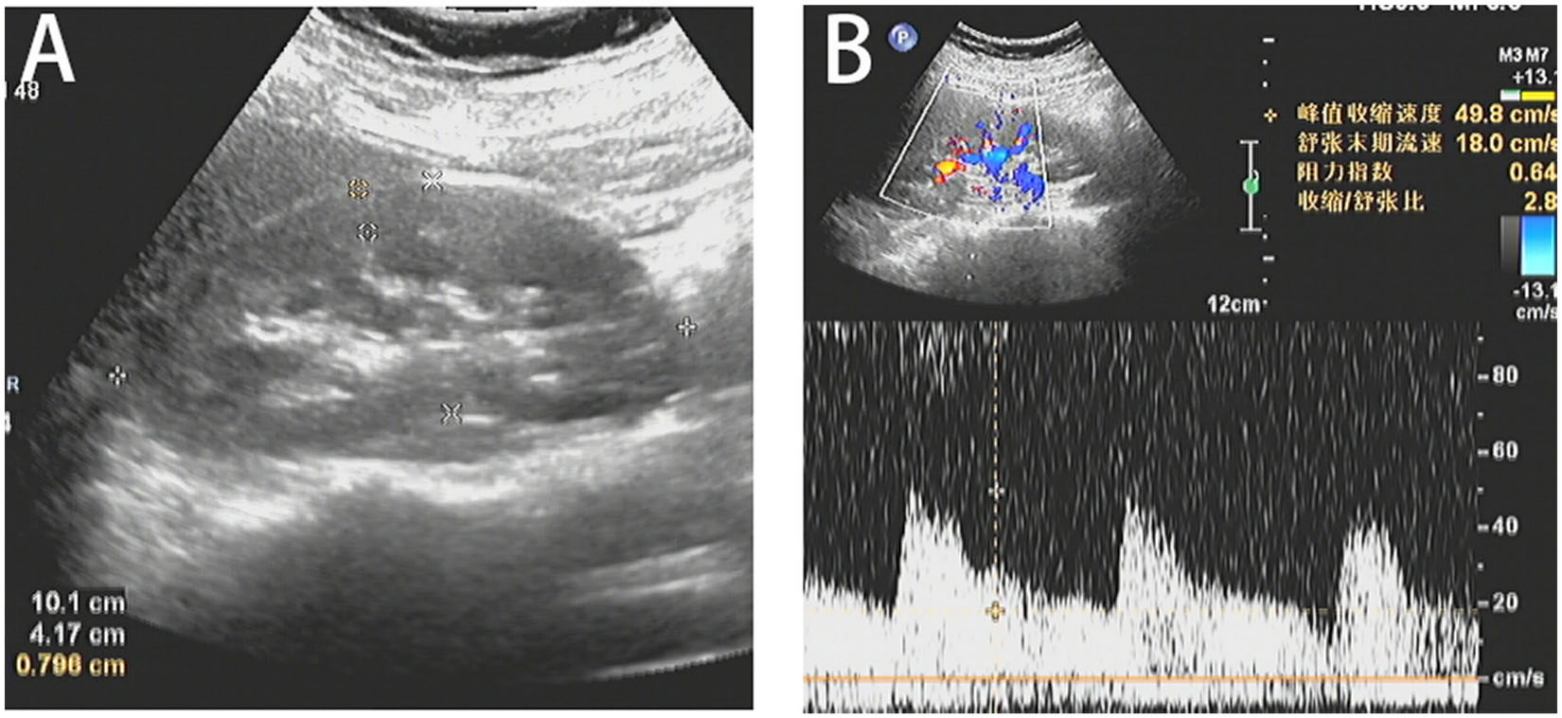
(A) The renal length, width, and cortical thickness of the right kidney measured; (B) the Doppler beam on the right MRA of PSV and RI.
